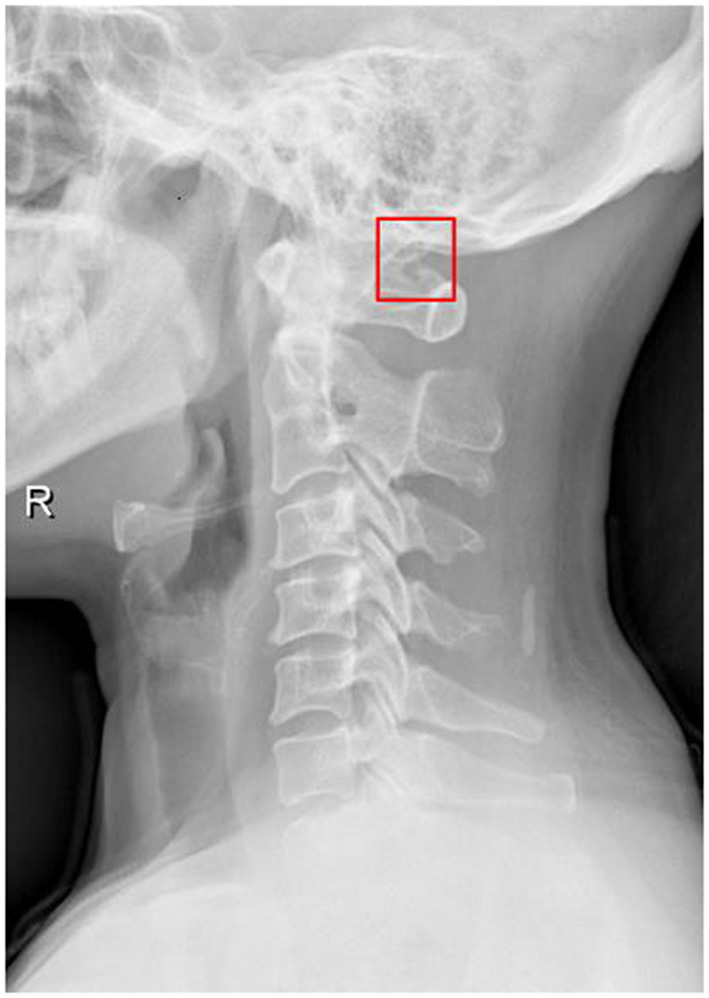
A 44-year-old female with migraine and normal neurologic examination. Lateral radiograph showed the right-sided partial ponticulus posticus, an anomalous bony bridge formed from the superior articulating surface of the atlas but not fused to the posterior arch of the atlas.
